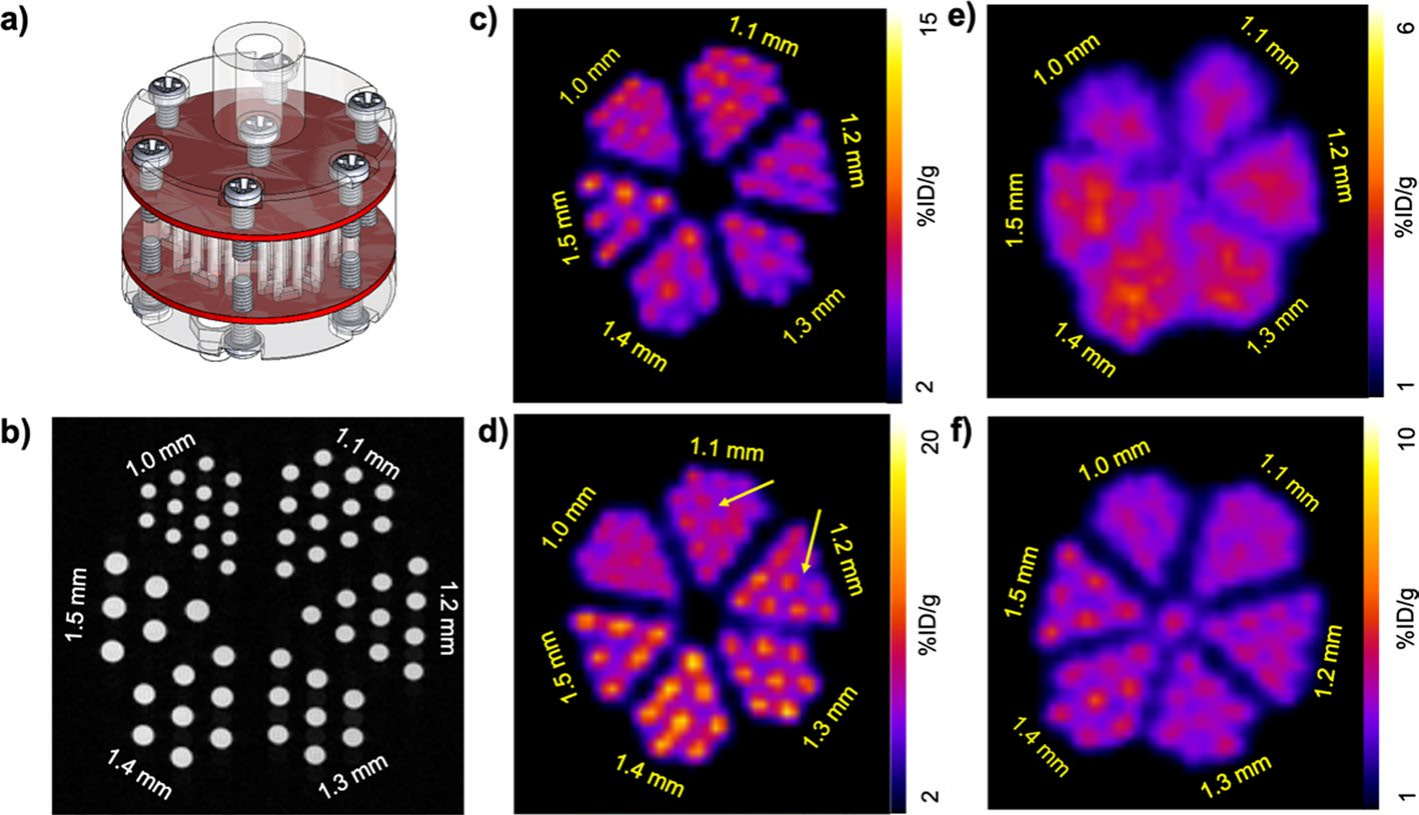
PET spatial resolution in the presence of the MRI instrument.*
